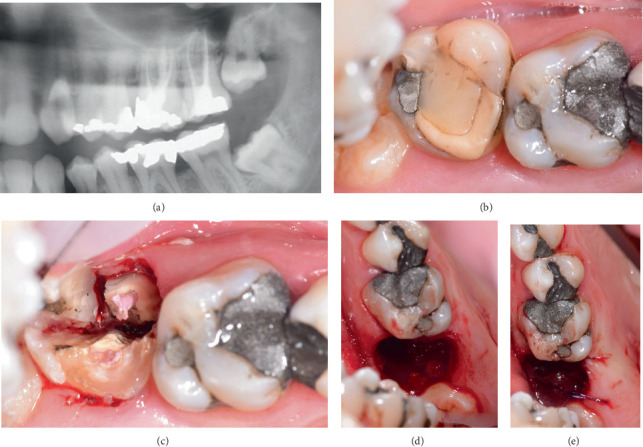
Preoperative situation. (a) The panoramic x-ray shows Tooth #15 with periapical radiolucency and a vertically placed wisdom tooth with suspected fused conical roots. (b) Clinical examination revealed a submucosal abscess with a fistula and Tooth #15 with insufficient postendodontic treatment and secondary caries. (c–e) The tooth was meticulously extracted after sectioning the roots.
